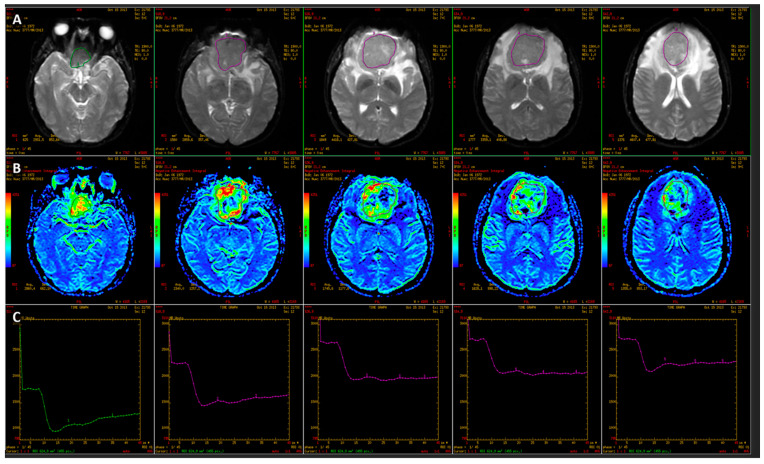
Perfusion-weighted imaging (PWI) analysis of a large parasellar meningioma. (A) Axial T2-weighted images showing the extent of the solid lesion with manually defined ROIs. (B) Corresponding perfusion maps demonstrating markedly elevated perfusion within the lesion, particularly in its anterior and peripheral regions. (C) Signal intensity–time curves derived from dynamic susceptibility contrast (DSC) perfusion sequences, showing a characteristic drop in signal intensity followed by partial recovery, consistent with high vascularity.
